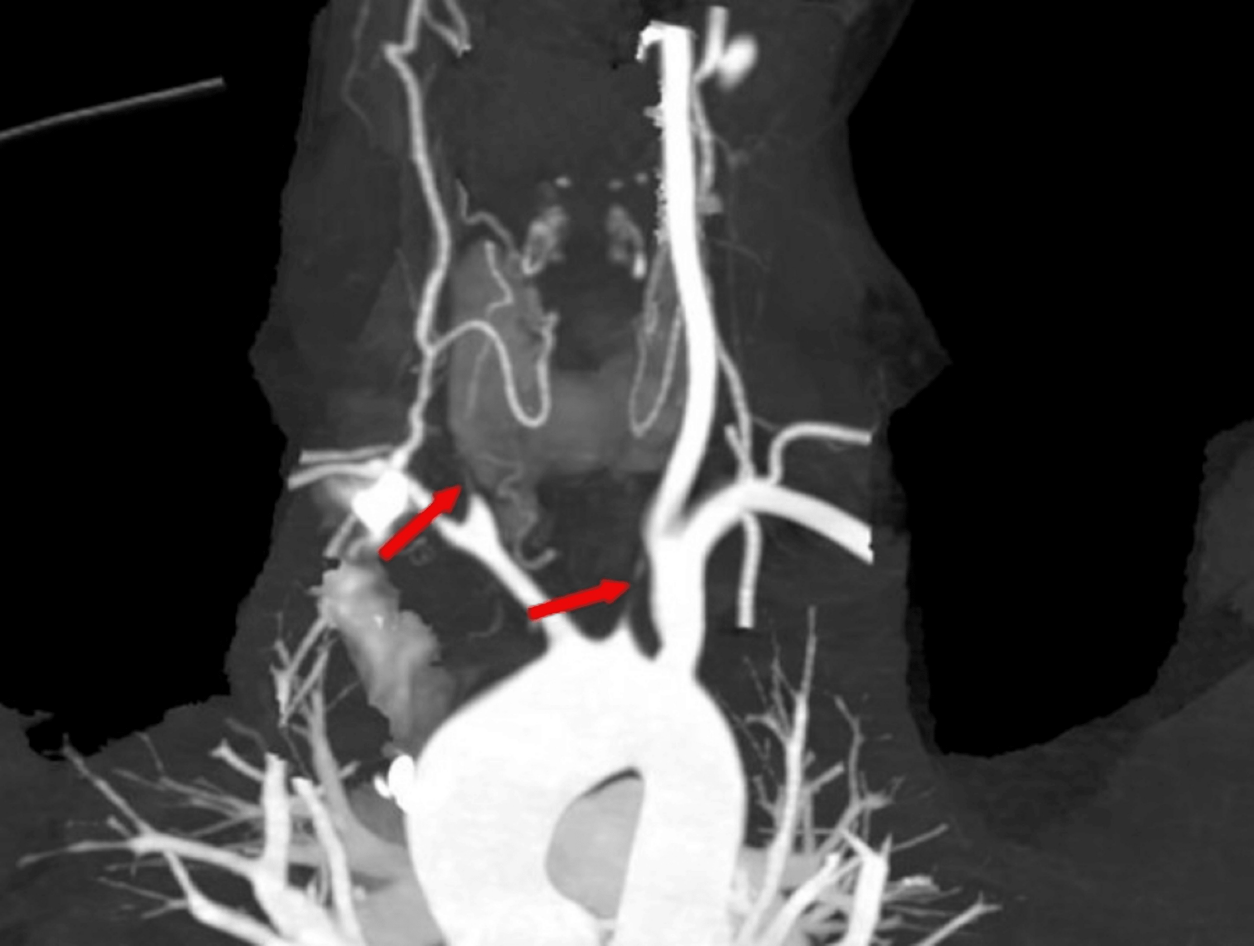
CT angiogram image showing bilateral common carotid artery (left and right CCA) stenosis with occlusion CT angiogram image shows the major vascular structures in the neck and upper thoracic region. The aortic arch is prominently seen at the bottom of the image with subtle diffuse arterial wall thickening of the proximal aortic arch. Minimal thickening is noted at origin of the left subclavian artery followed by normal caliber of rest of the artery and left vertebral artery. There is significant circumferential wall thickening of the left common carotid artery producing multifocal occlusion in proximal half. There is significant luminal narrowing (>50%) noted in distal half of left common carotid artery (arrow) up to bifurcation due to thrombus formation. The right common carotid artery shows near-complete occlusion throughout its length, while the innominate artery and right subclavian artery exhibit over 50% narrowing (arrow). The proximal right vertebral artery is not visualized with distal segment showing slightly reduced caliber and normal contrast enhancement. Mild thickening of the left common femoral artery and enlarged left external iliac nodes was also noted.
